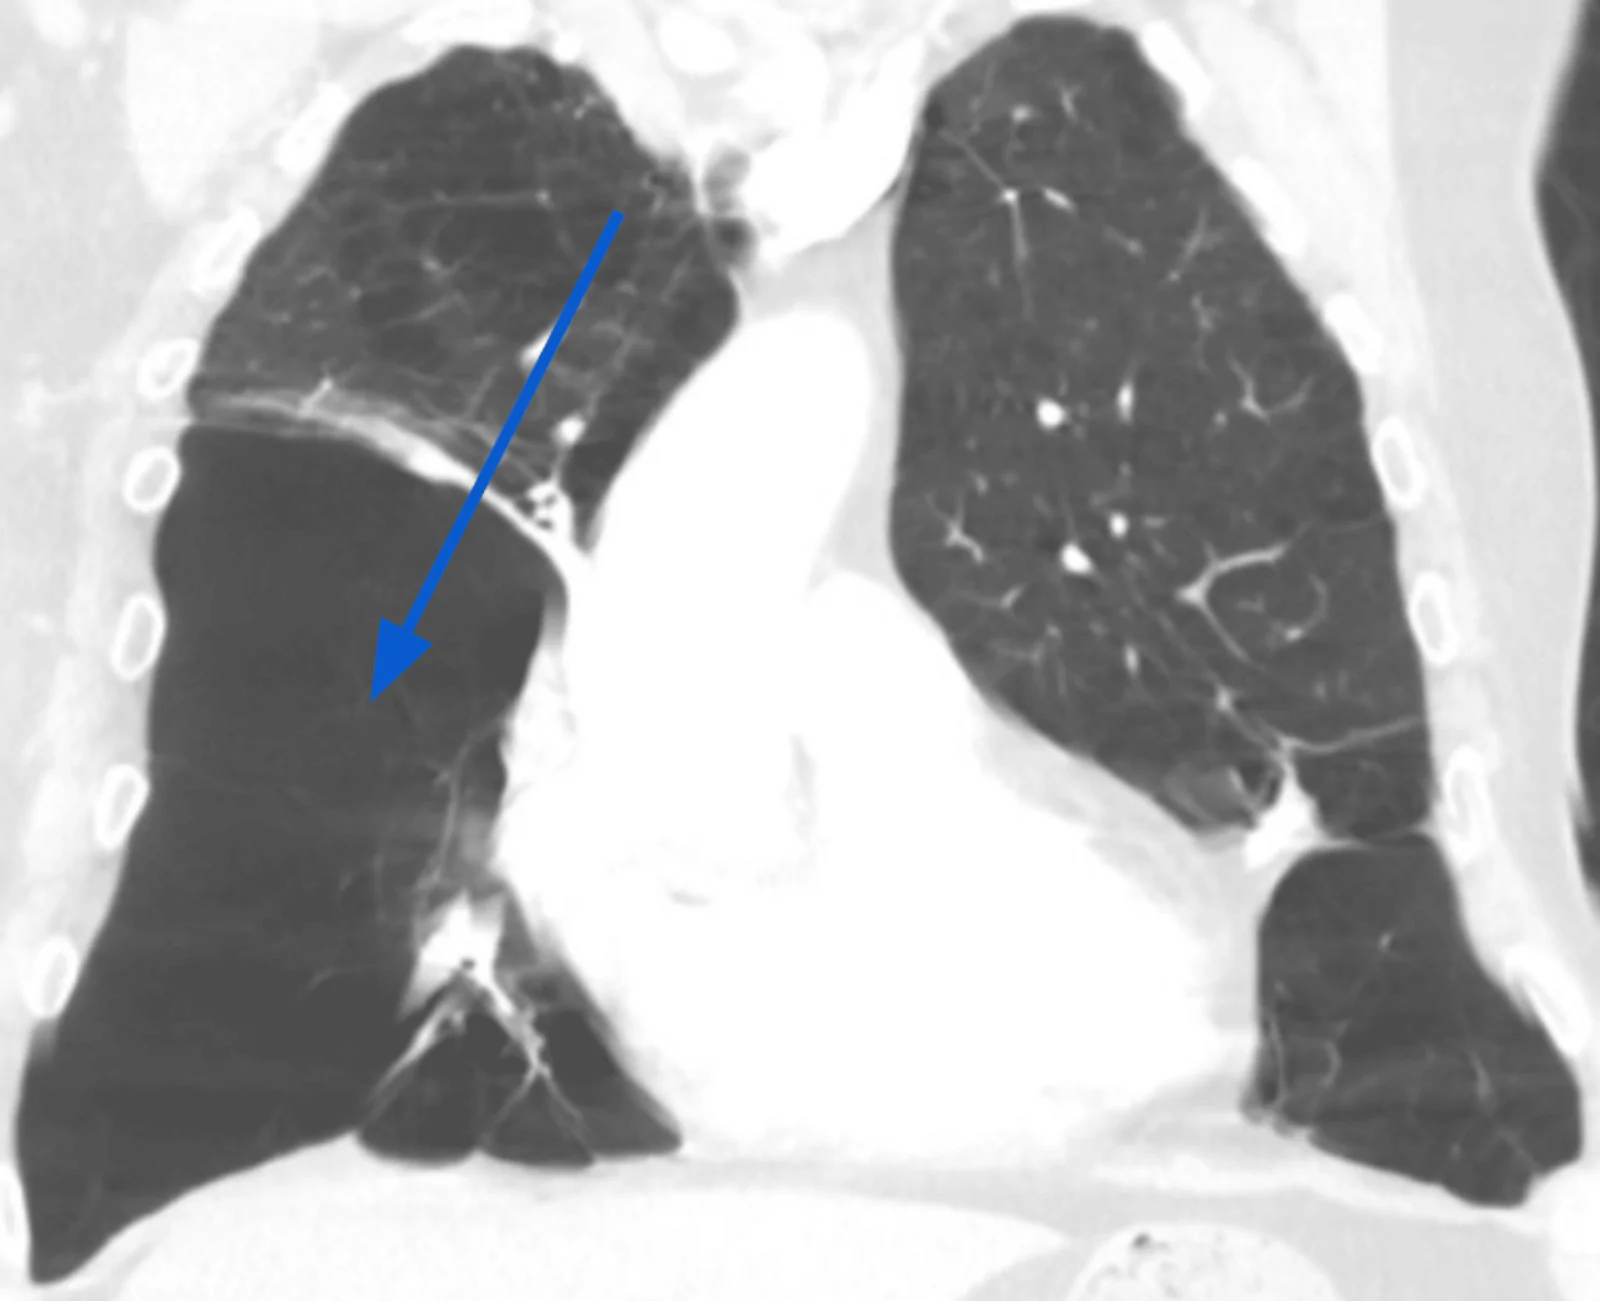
Coronal computed tomography obtained one month before surgery. The blue arrow identifies the large right-sided bulla.
